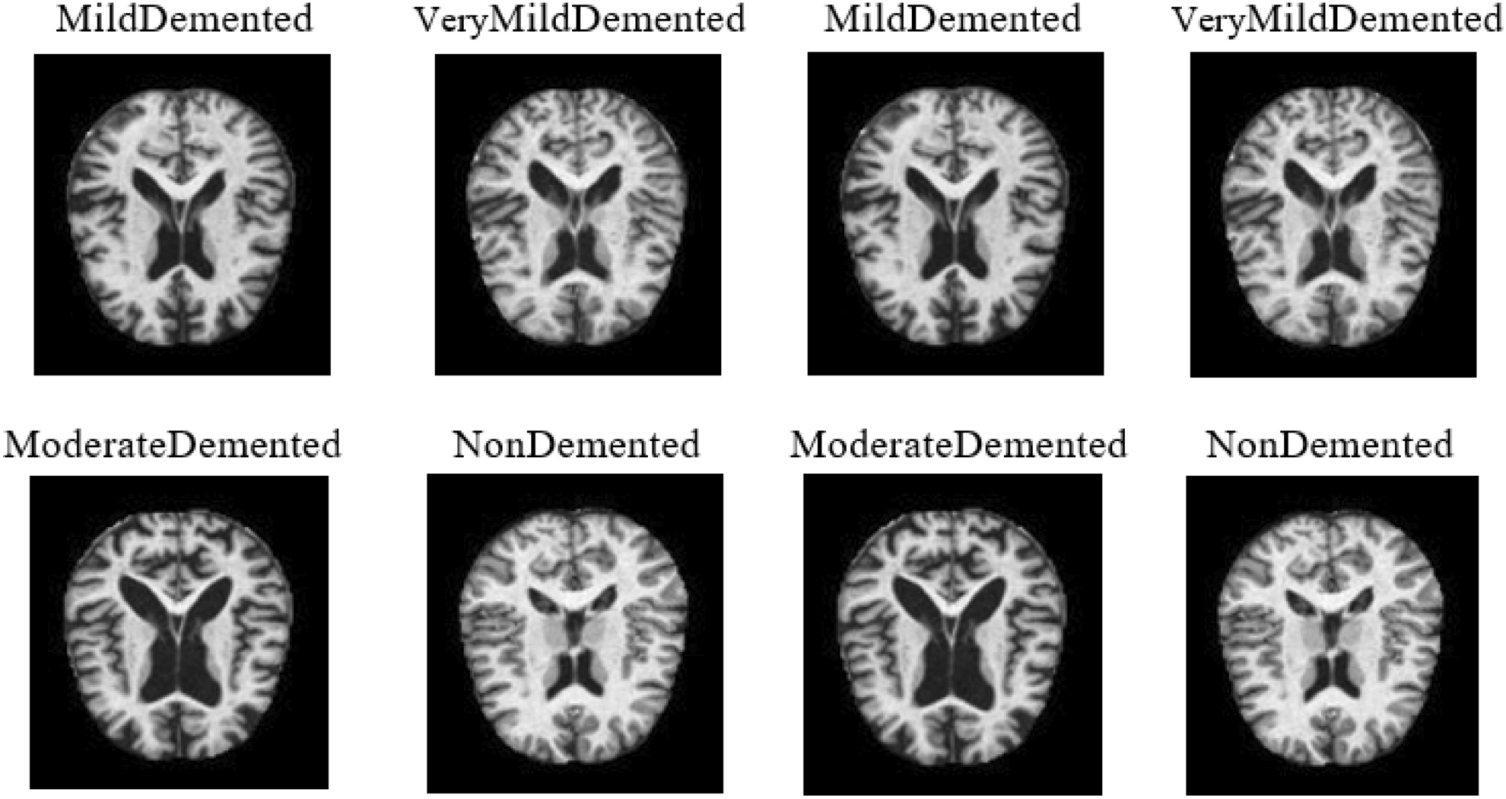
Some Samples of MRI images are utilized.
